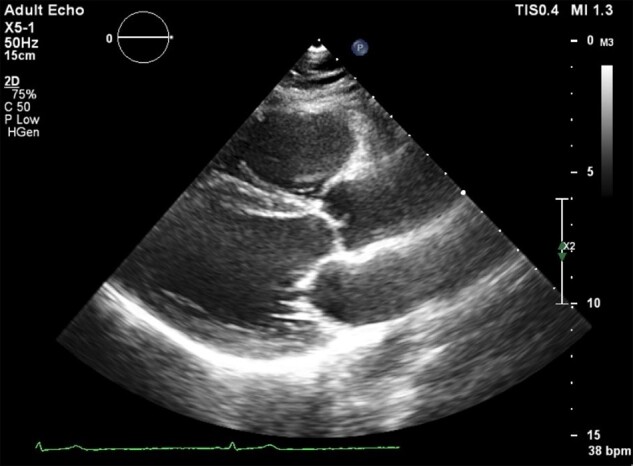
Echocardiography section from parasternal long-axis view showing a mild concentric hypertrophy of the left ventricle.
